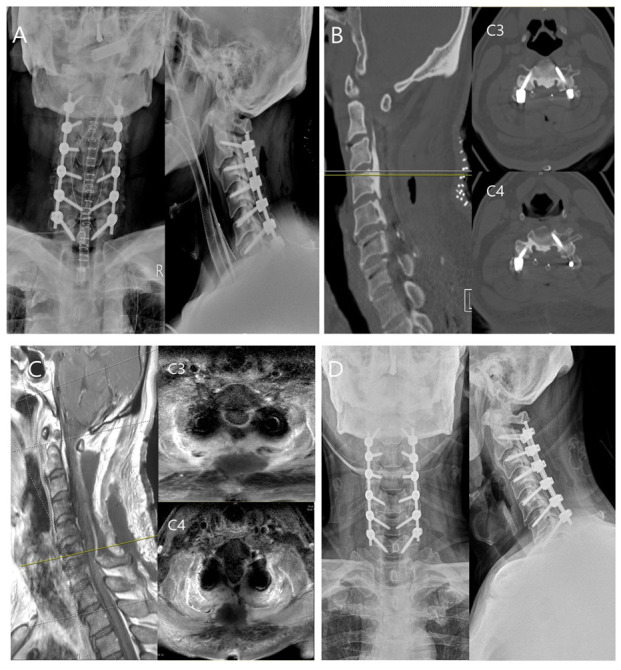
A 63-year-old male presented with reduced sensation below T4 and bilateral hand clumsiness after falling down stairs. Preoperative computed tomography showed K-line (-), C2–C7 mixed-type ossification of the posterior longitudinal ligament, with spinal canal encroachment. Urgent surgical treatment (decompressive laminectomy and posterior fusion with pedicle screws at C2–C7) was performed, and the immediate postoperative X-ray is shown in (A). However, the patient developed a continuous high fever and significant CRP elevation on postoperative day 4; CT images showed no bony destruction, but swelling and gas formation of soft tissue (B). Magnetic resonance T1 images showed a fluid collection around the deep soft tissue, consistent with an acute postoperative infection (C). I&D surgery using a toothbrush was performed 10 days after fusion surgery. This resulted in infection control and symptom improvement. The patient was discharged 1 month after the I&D surgery. One-year postoperative X-rays showed successful infection control, good alignment, and bony fusion (D). The patient provided informed consent to publish all of his clinical images.
